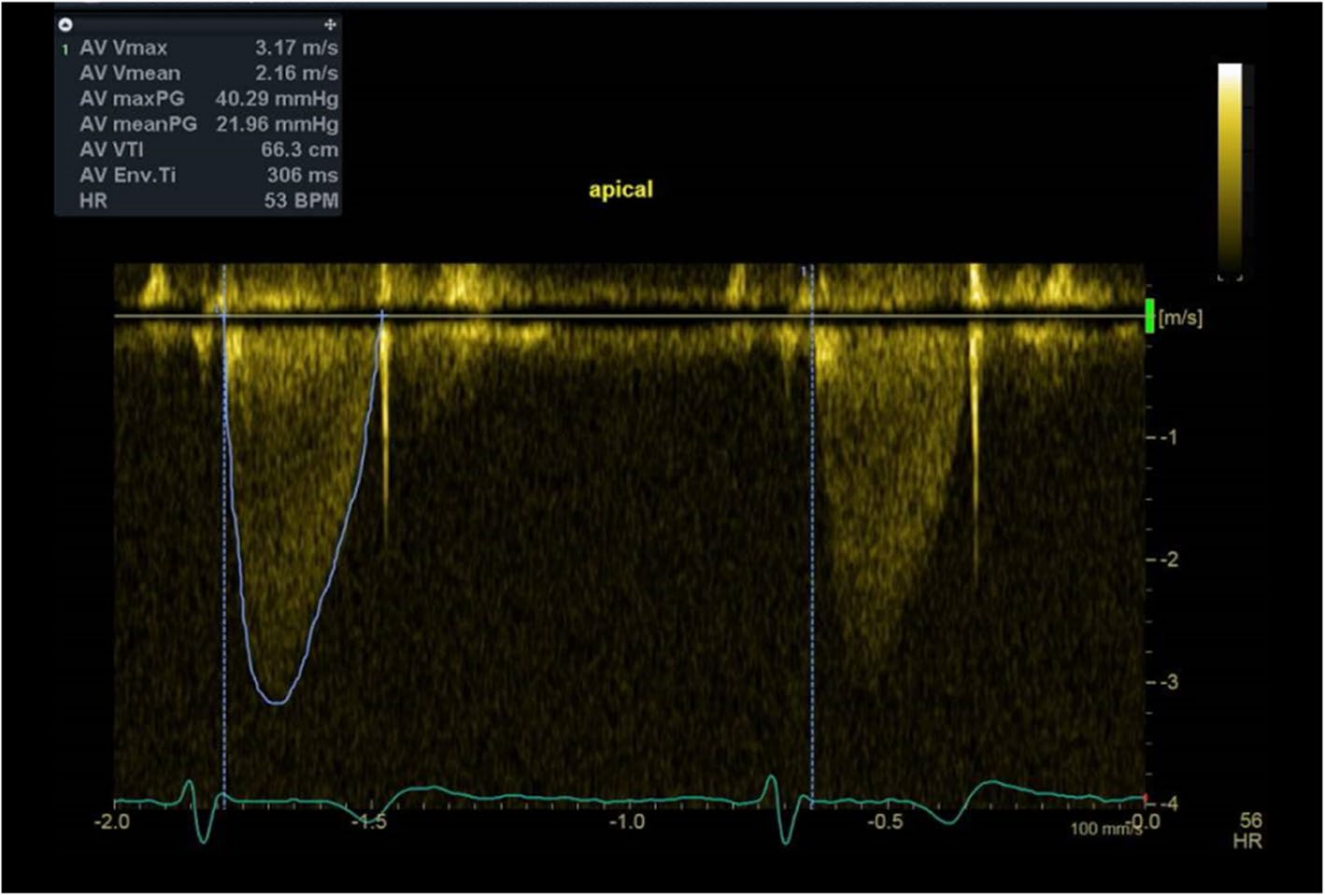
Transthoracic echocardiography at presentation. Raised transaortic gradient is likely underestimated by low quality Doppler signal of transthoracic echocardiography
